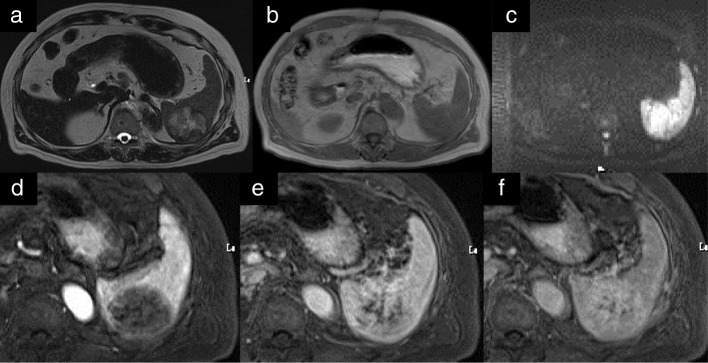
a-c: The tumor has high intensity on magnetic resonance imaging (MR) T2WI, equivalent to or slightly lower than that in MRI-T1WI, and no decrease in diffusion. d-f: The tumor is multinodular with a low-signal spoke-wheel sign in the margin, enhanced gradually in the dynamic study
